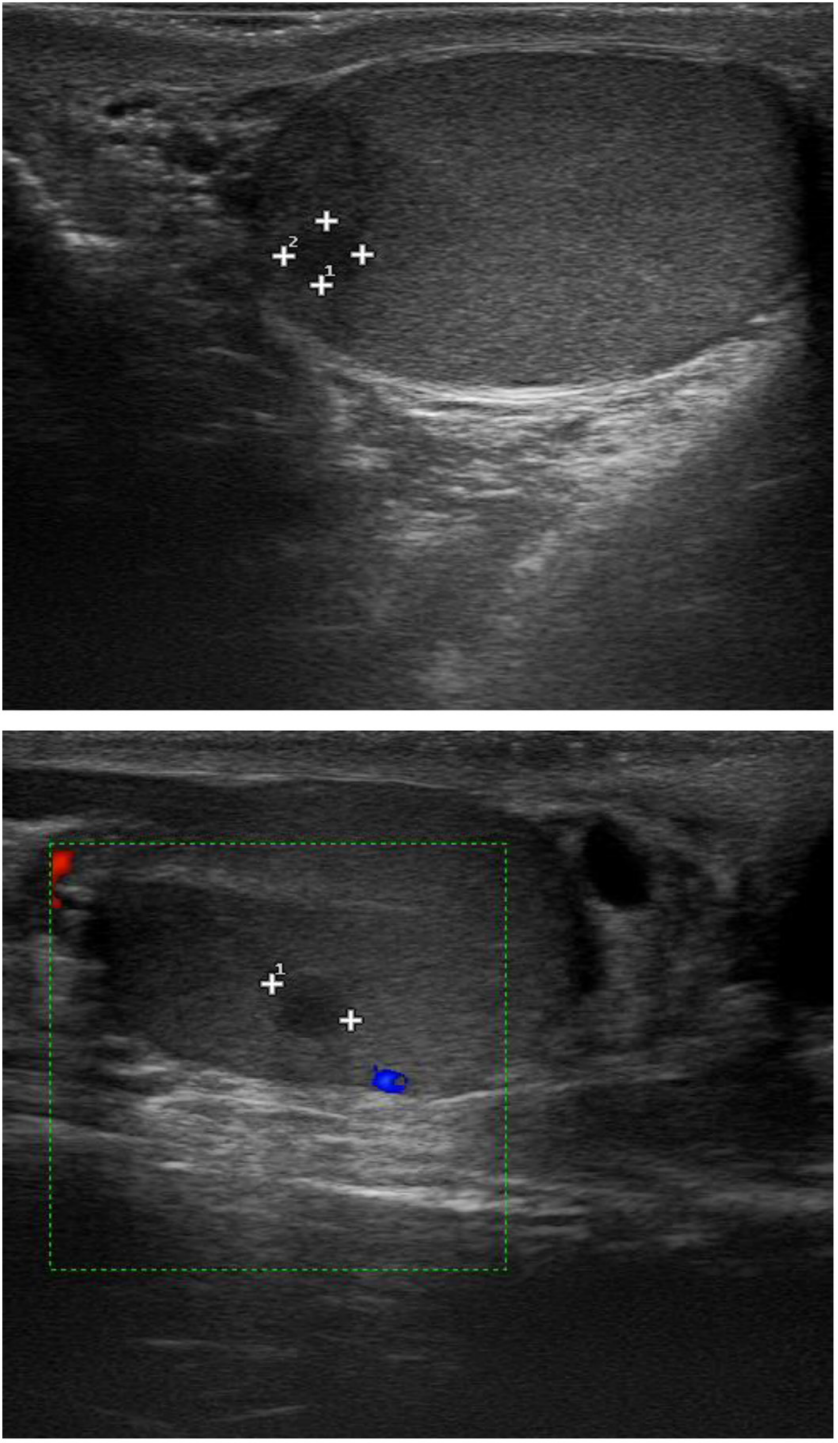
Ultrasound imaging. Hypoechoic lesion of 5 mm in the middle part of the right testis, without a clear vascular pattern.
